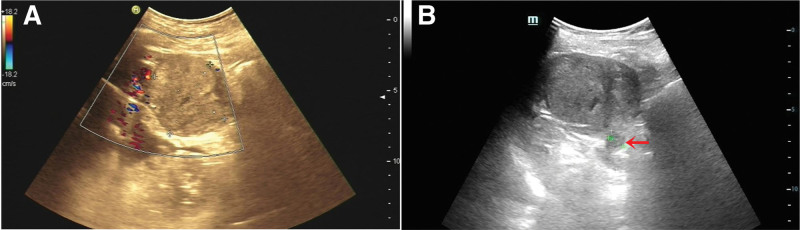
(A) Color Doppler ultrasound indicated a solid mass with blood flow in the middle part of the left kidney. (B) A hypoechoic mass in renal vein was indicated by the arrow.
